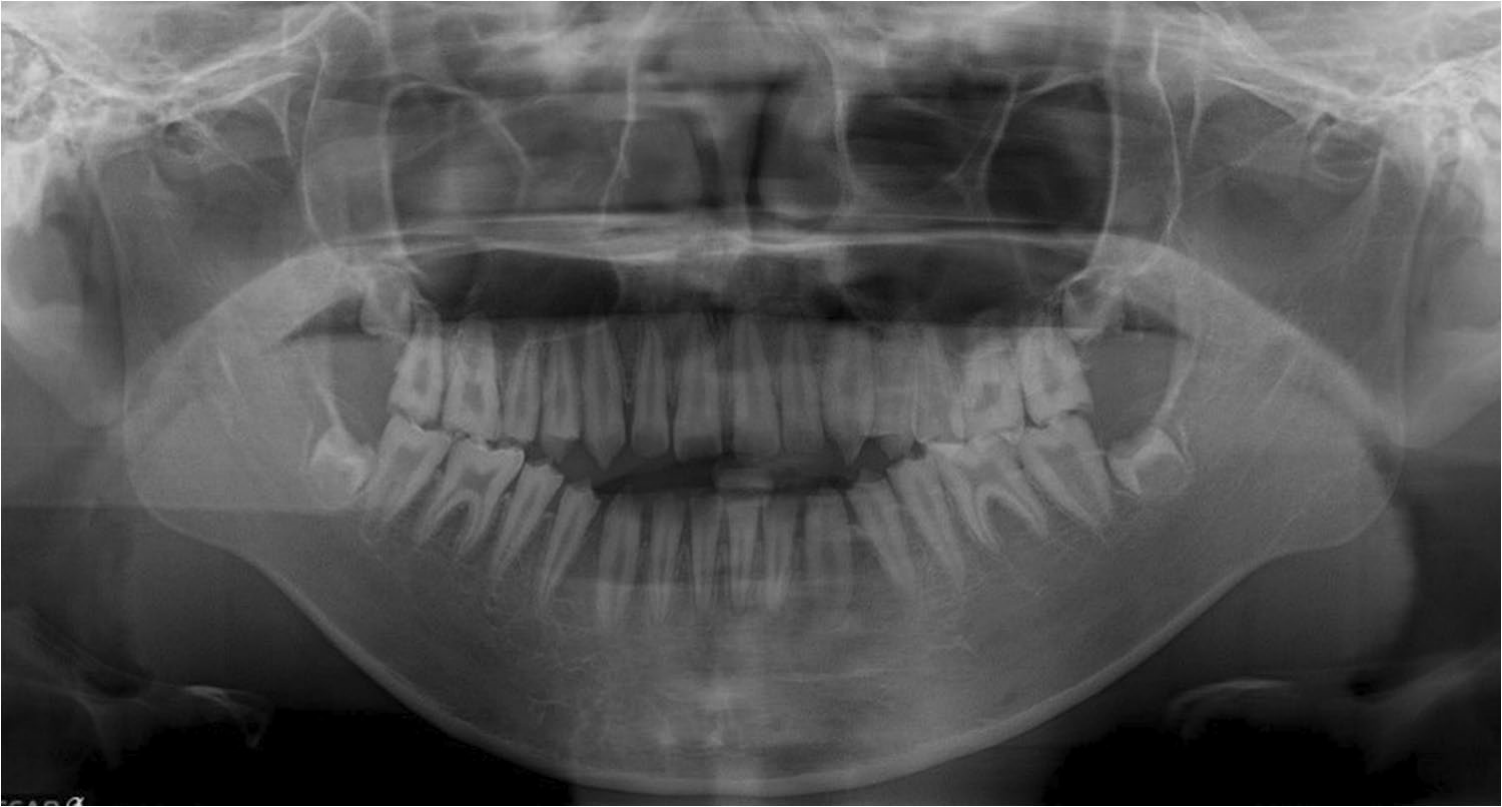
Panoramic radiograph of the individual (4–7) in the family. In the panorama radiograph, enamel was hardly observed, and a very thin layer of enamel was observed only in some teeth. A space between the teeth was also observed because of the lack of enamel formation
